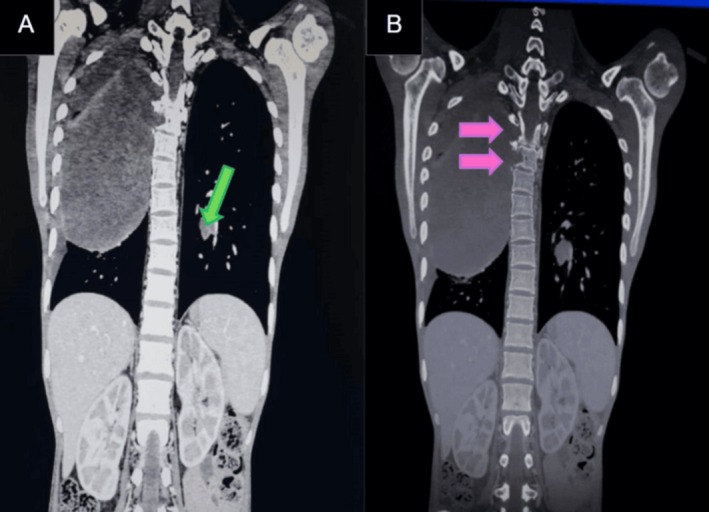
(A) Coronal CT soft tissue window image showing a similar‐looking soft tissue nodule in the contralateral lung (green arrow). (B) Coronal CT bone window image showing vertebral erosions (pink arrows).
